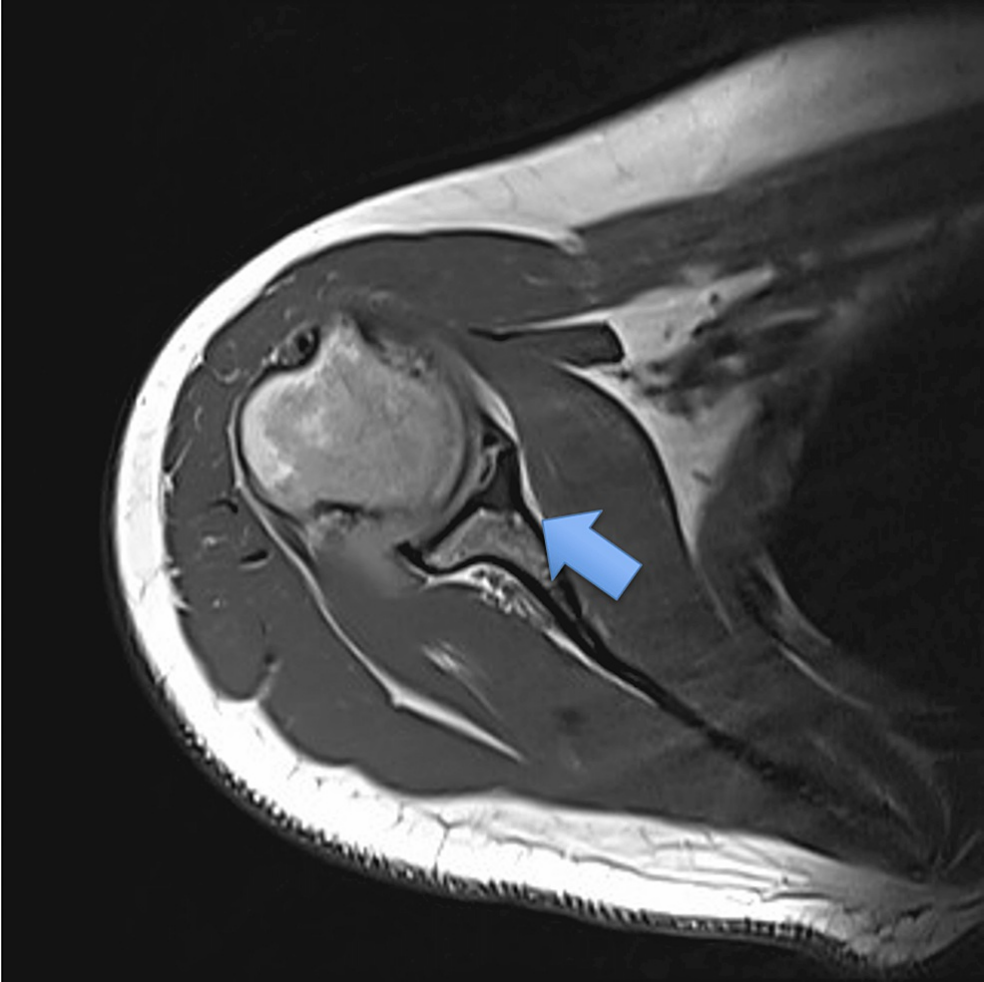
Axial view of MRI shows the glenoid cyst.
